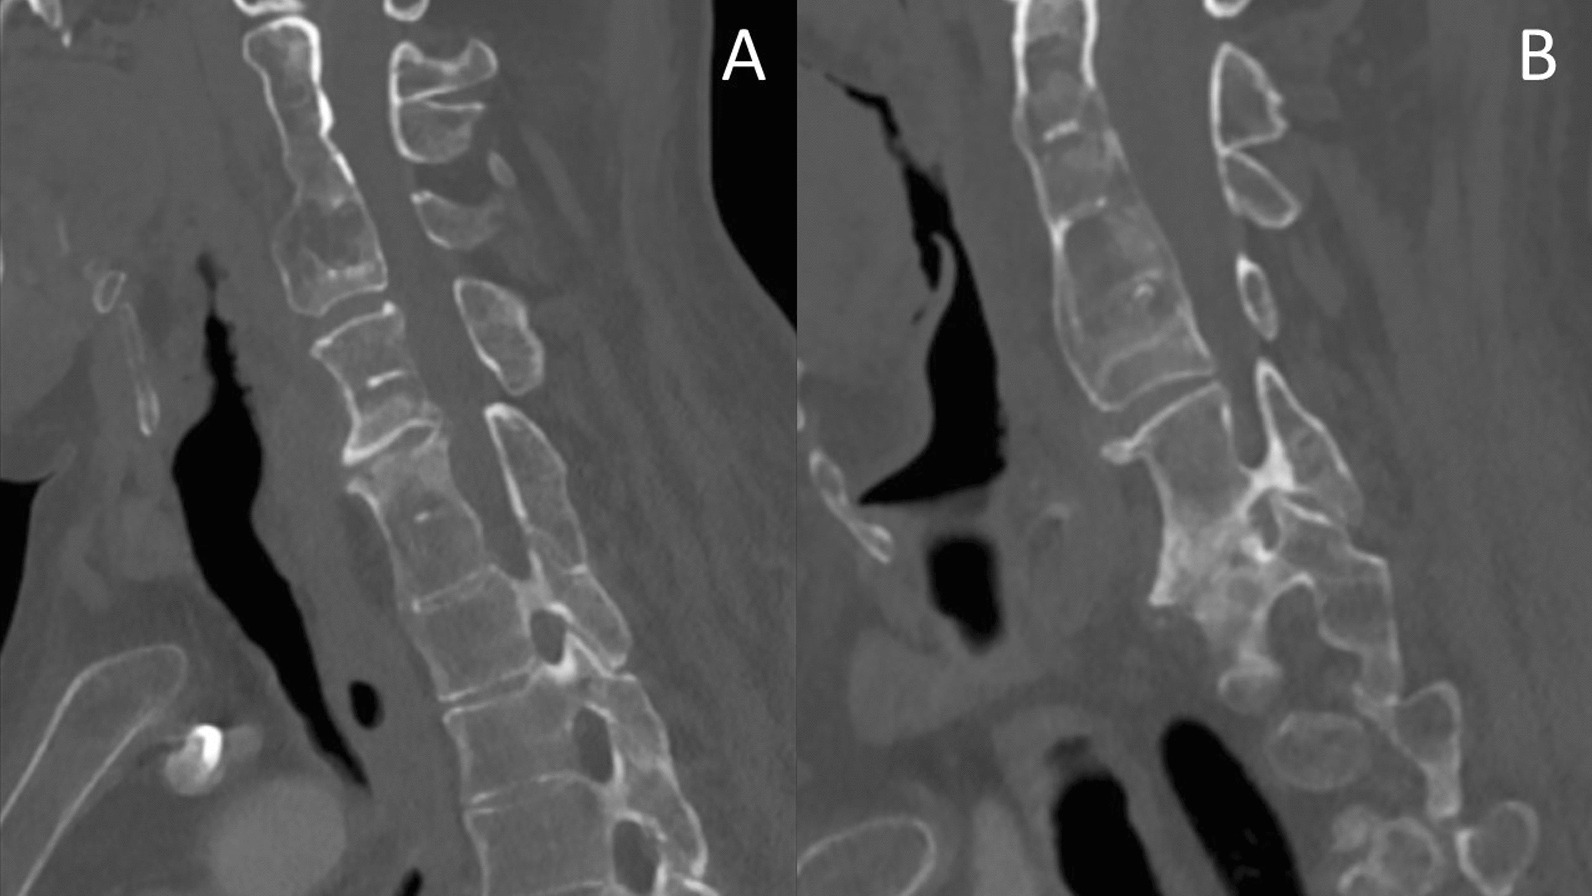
Cervical spine computed tomography scan showing congenital cervical fusion and cervical stenosis. A sagittal view showing congenital fusion at C5-C6 and C7-T2 levels. B sagittal view showing congenital fusion at C2-C4 levels
